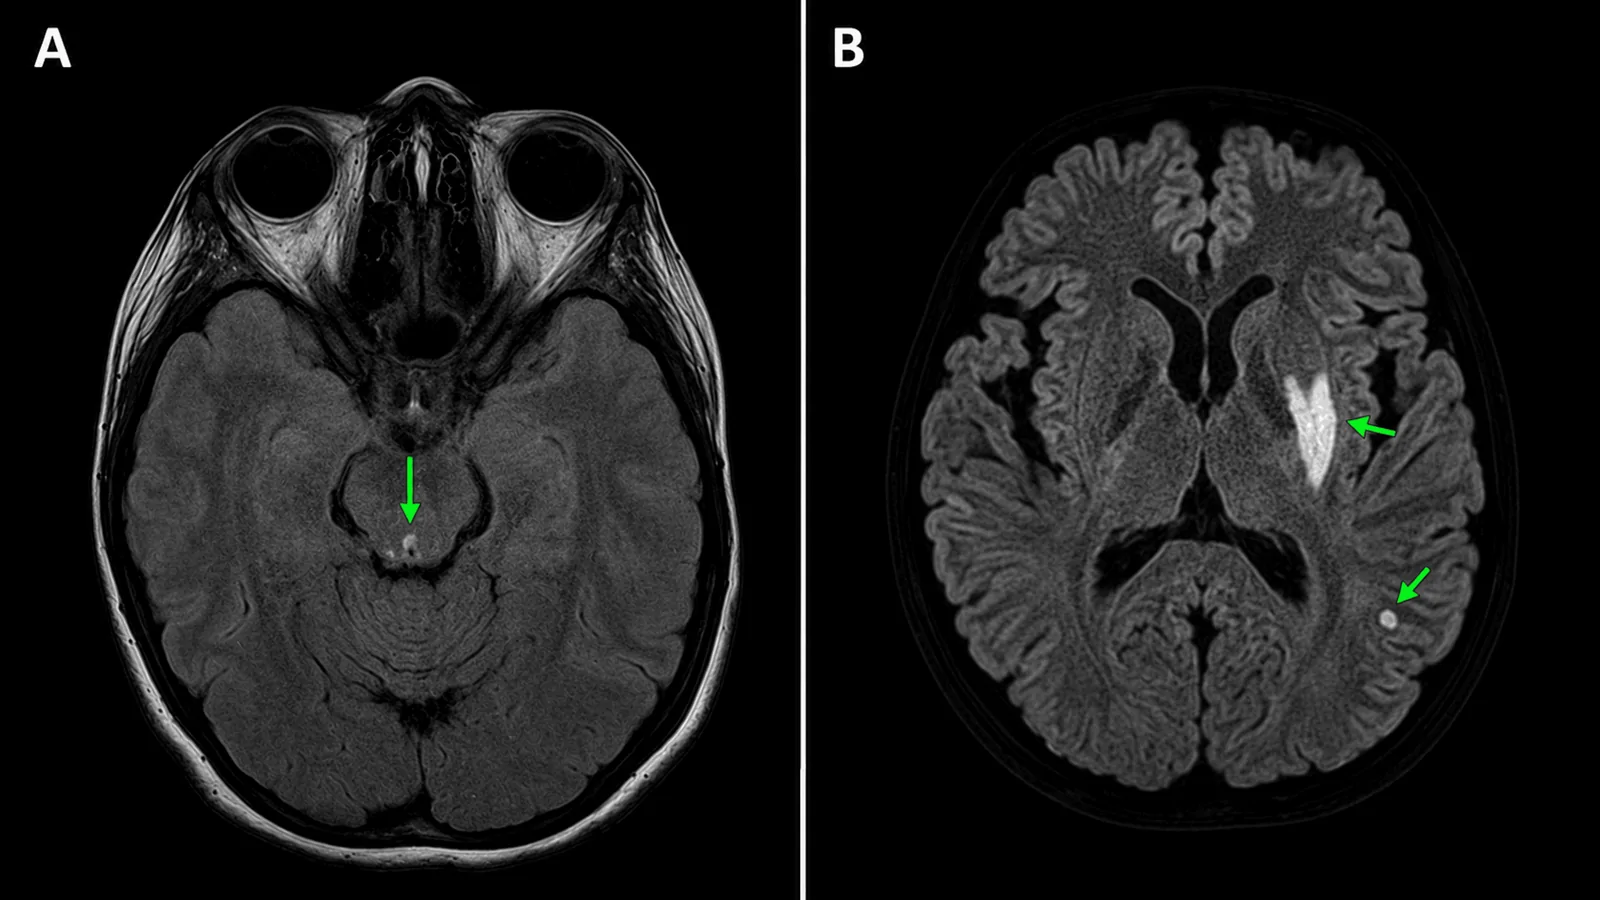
Brain magnetic resonance imaging. A: Axial FLAIR image demonstrating a hyperintense lesion in the pons (arrow). B: Axial diffusion-weighted imaging (DWI) demonstrating multiple acute ischemic infarcts involving the left corona radiata and left temporal lobe (arrow)
